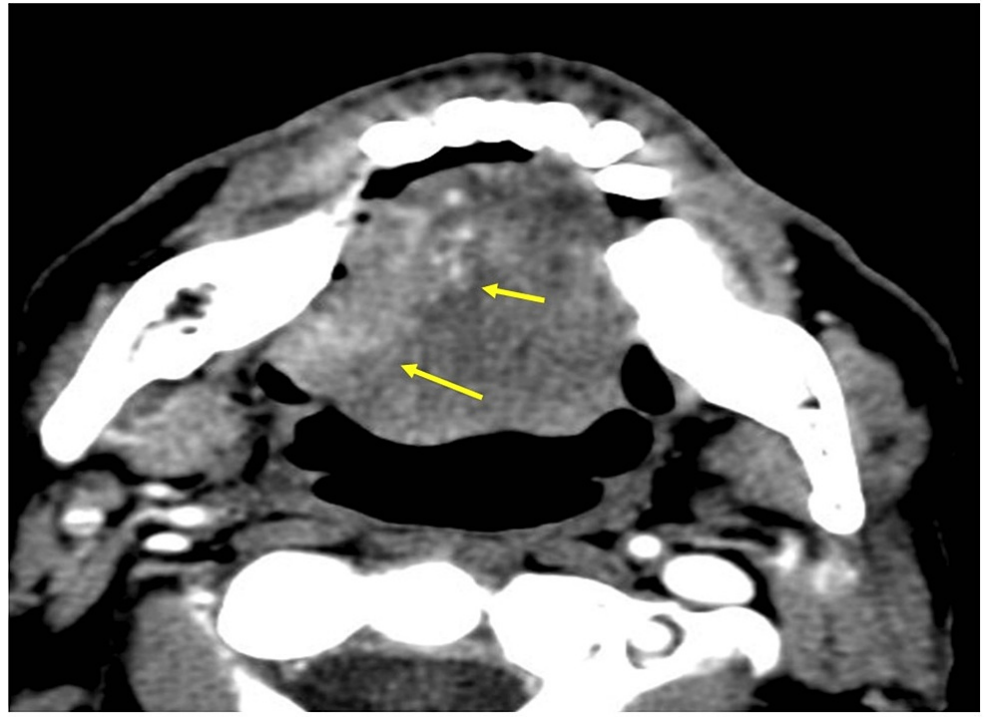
Preoperative contrast-enhanced CT shows a large SCC of the right lateral tongue (yellow arrows)
